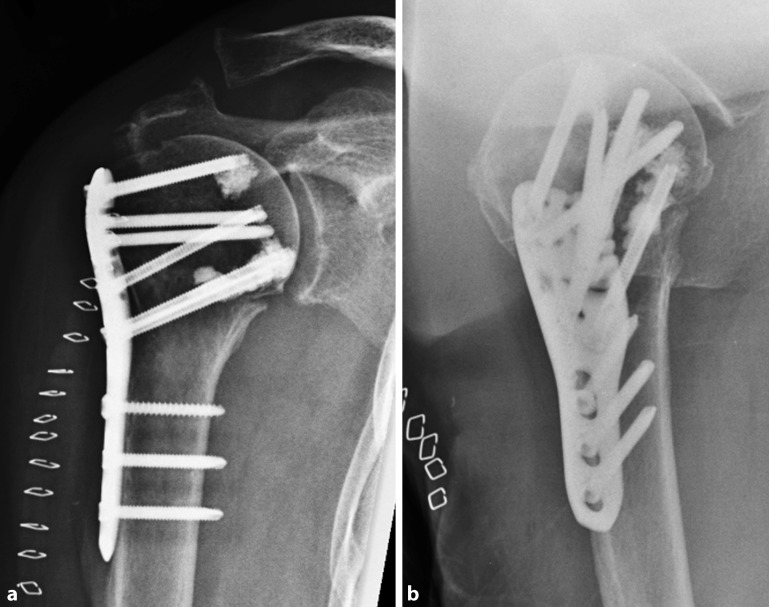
Postoperative radiographs of an 87-year-old female patient after locked plating of a proximal humeral fracture (see Fig. 1) with additional cement augmentation of the anterosuperior and inferior humeral head screws (a anteroposterior, b axillary view)
